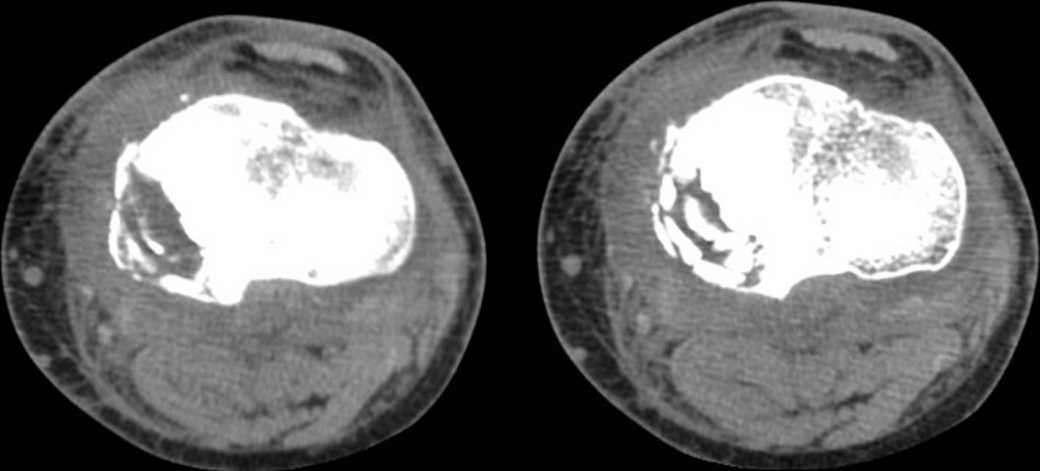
Preoperative CT scan of the left knee confirming the diagnosis of a Schatzker type IV fracture of the proximal tibia
